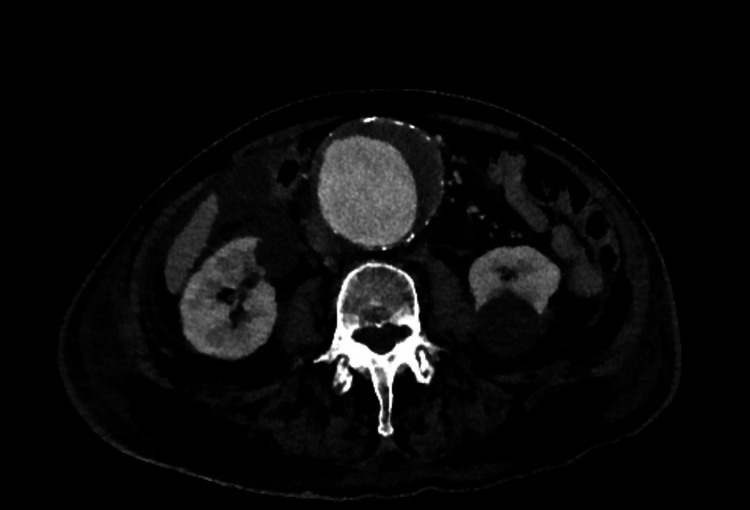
CT angiogram showing an axial view of a large 7.5 cm infrarenal abdominal aortic aneurysm with incidental bilateral renal cysts.
